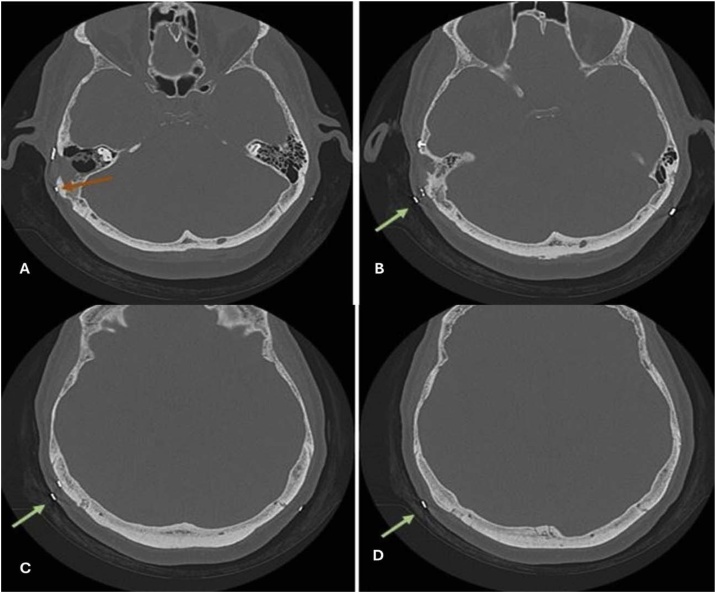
Image-guided implant placement estimation conducted in subject #28. Computed Tomography (CT) showing (A) The fixation screw in the patient's skullcap, at Points 1 and 2; (B) Point 1 showing proximity to veiled mastoid cells; (C) Point 2 showing increased bone thickness; (D) Point 3 showing increased bone thickness. Points 1 and 2 were excluded and Point 3 was selected for implant placement.
